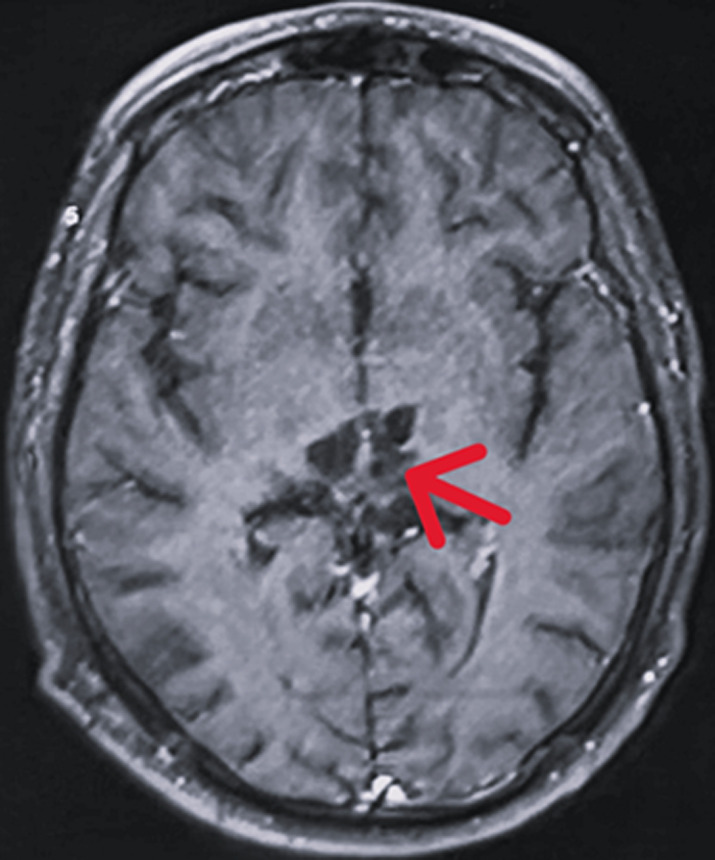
contrast-enhanced brain magnetic resonance image showing a thalamo-peduncular lesion: axial T1-weighted post-contrast magnetic resonance image demonstrating a thalamo-peduncular mass with heterogeneous patchy enhancement and multiple small cystic components (red arrow)
